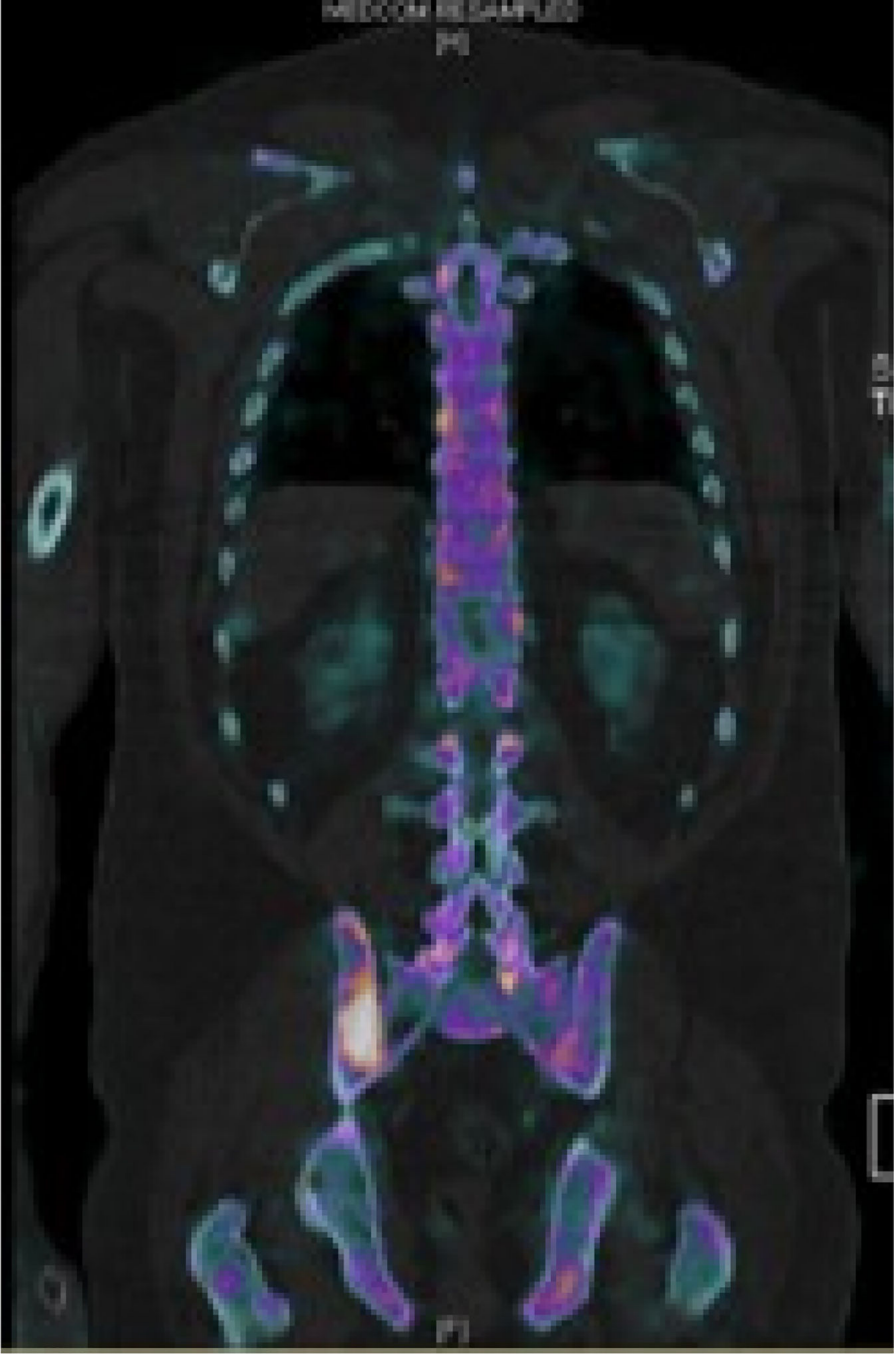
Nuclear medicine bone scan with 99mTc-hydroxymethylene diphosphonate (HMDP) and SPECT showed multiple areas of uptake concerning for malignancy. Uptake shown in right posterior iliac bone.
